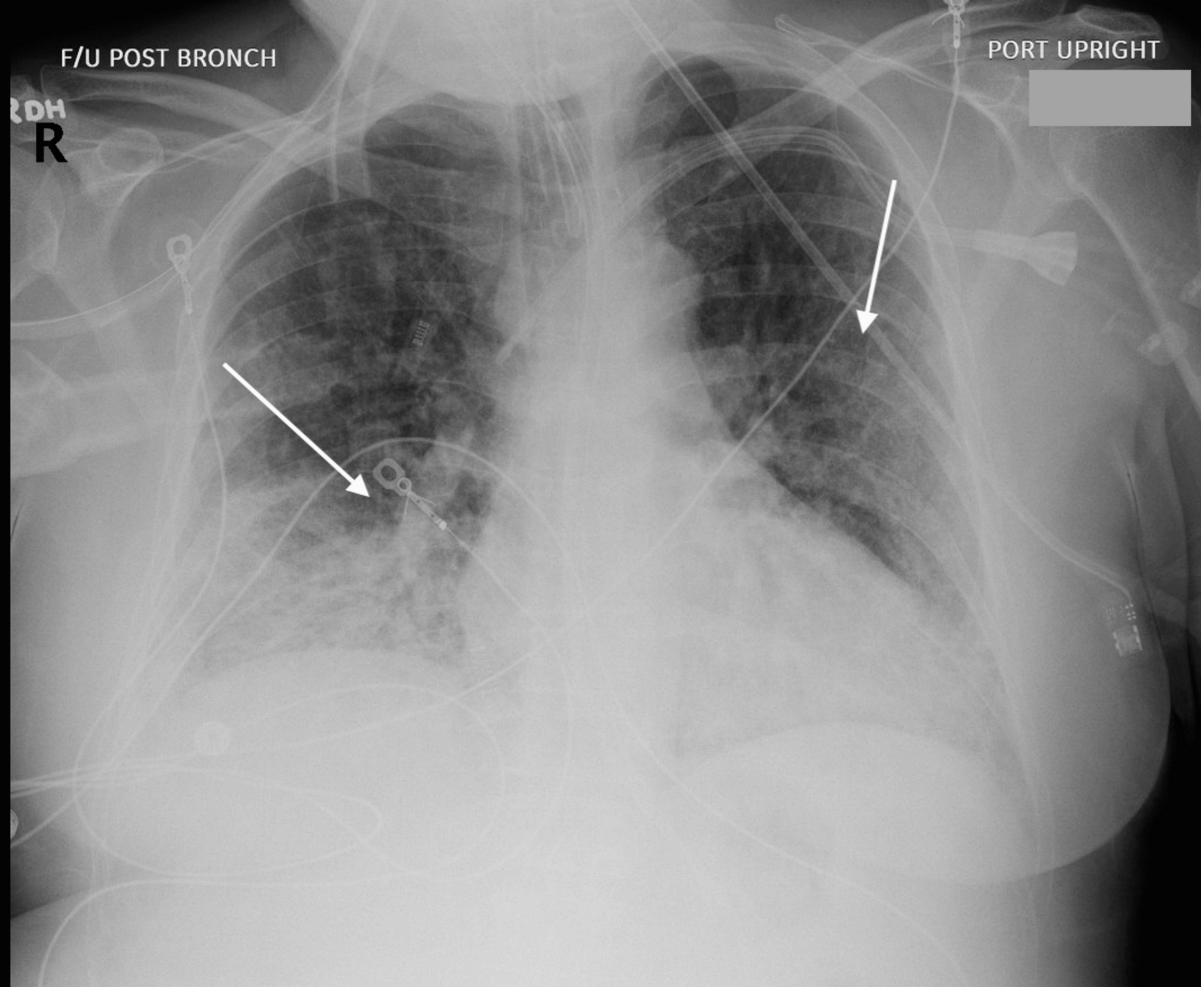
The chest radiograph following bronchoscopy demonstrates bilaterally increased interstitial markings, consistent with progression of respiratory distress.
